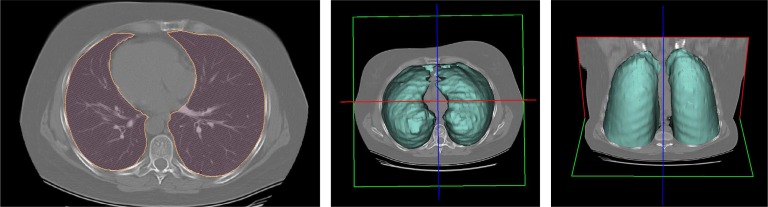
(Left) Axial single chest CT and its (middle and right) FC delineation correspondence are seen.
